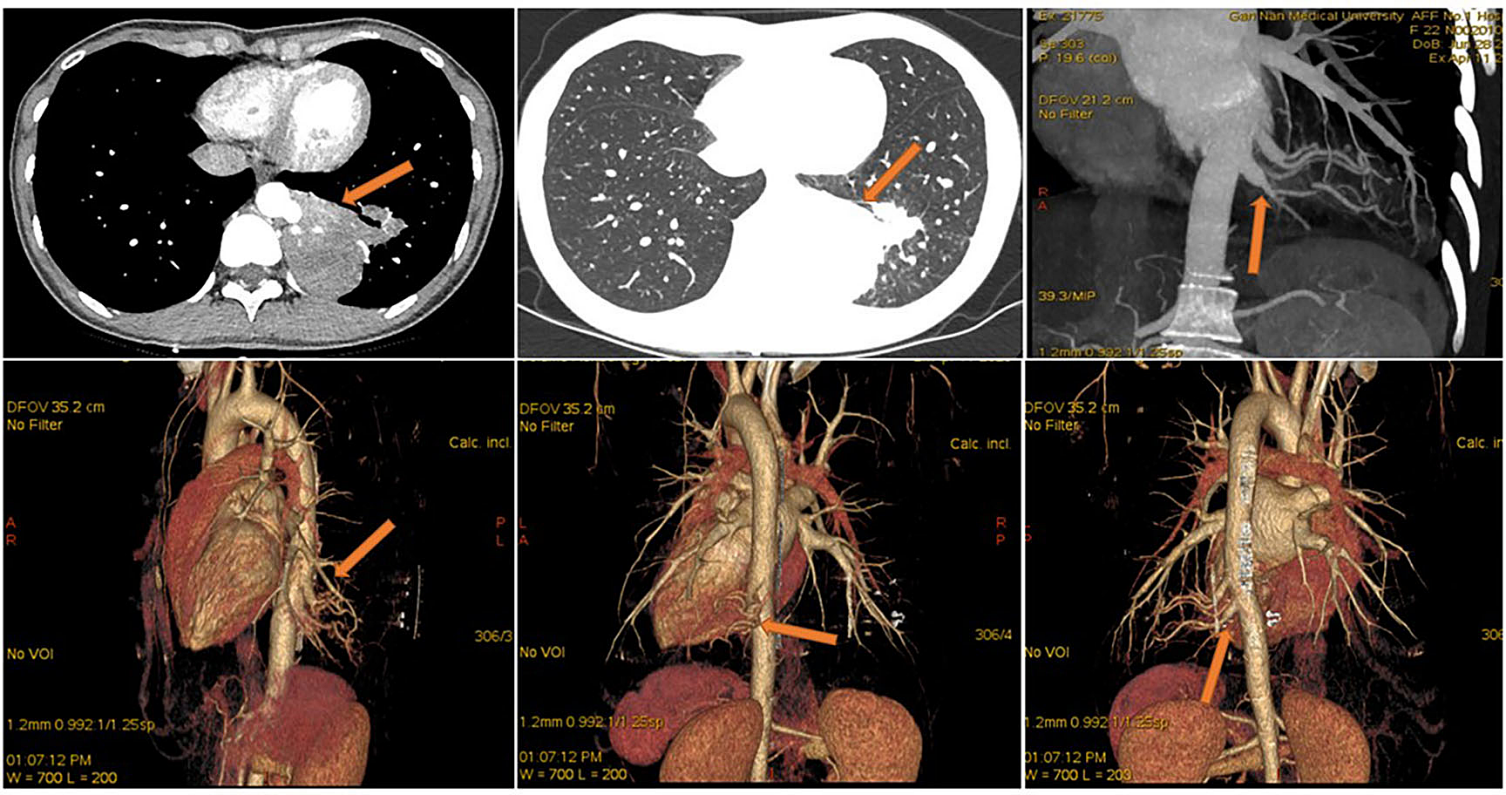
The thoracic aorta sent out three branch arteries into the lesion. Enhanced chest CT combined with three‐dimensional vascular reconstruction shows a mass‐like density shadow in the lower lobe of the left lung, uneven enhancement, and a strip shadow around it. Three branch arteries from the thoracic aorta enter the lesion.
